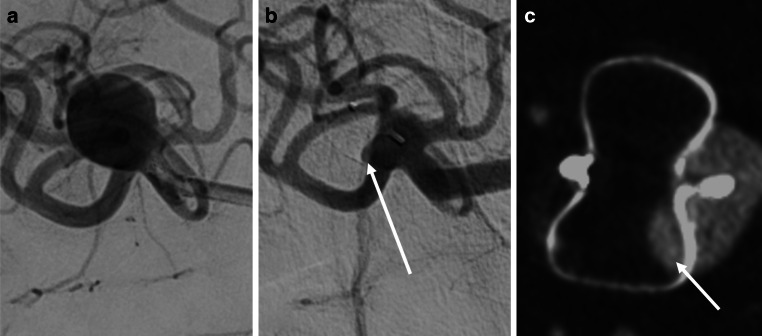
A representative case of a middle cerebral artery bifurcation aneurysm treated with the Woven EndoBridge (WEB) device. a Digital subtraction angiography (DSA) prior to WEB placement. (b) DSA and (c) intravenous cone-beam computed tomography (IVCBCT) performed at the 6‑month follow-up demonstrate a BOSS of 1^33%^ (minimal contrast opacification within the WEB device without neck remnants)
